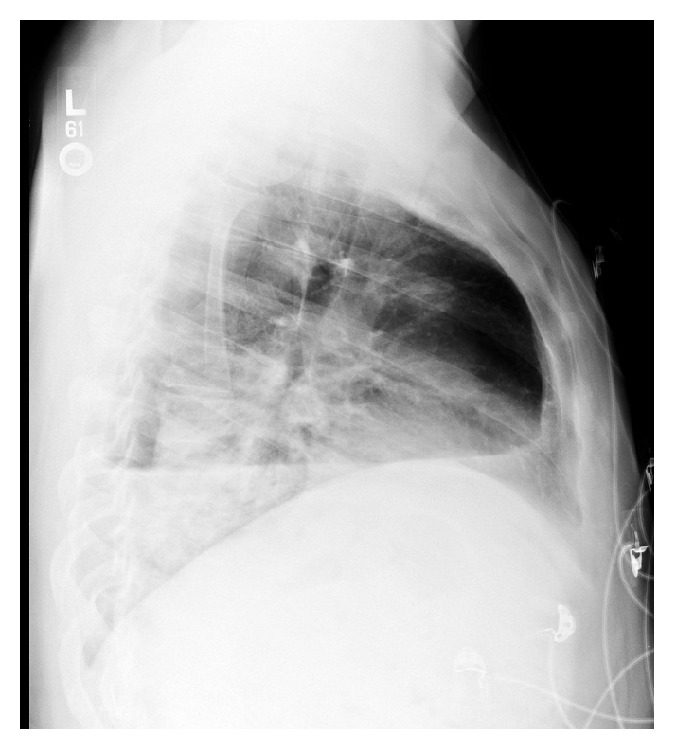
Chest X-ray on representation demonstrating pyopneumothorax.
